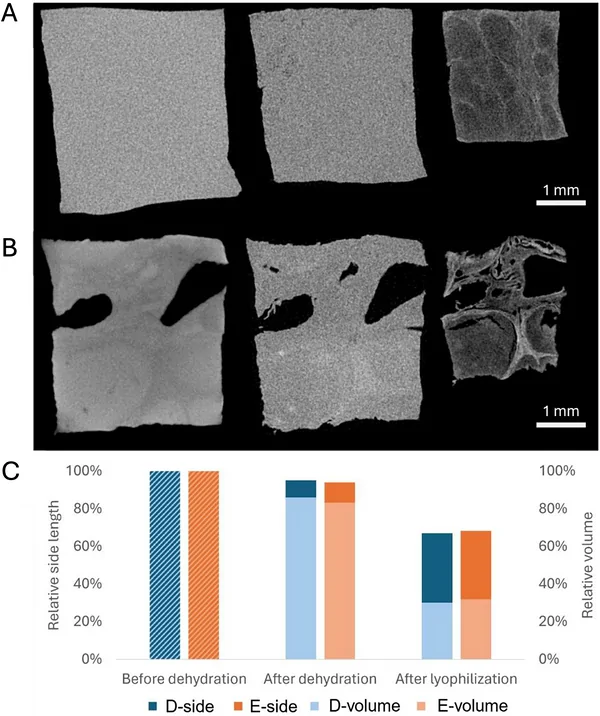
Process‐related shrinkage of the liver samples. (A) shows CT scans of the same sample undergoing protocol E before dehydration (left), after dehydration (middle), and after lyophilization (right). (B) shows the same scanning time points for a sample undergoing protocol D. (C) shows the calculated sample shrinkage at different time points. The chart shows the changes in total volume (light color) and average side length (dark color) for the respective samples undergoing protocols D (blue) and E (orange). *Source*: own.
